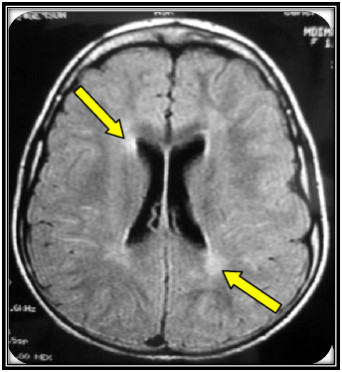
Brain MRI of the patient with late infantile form of MLD.
